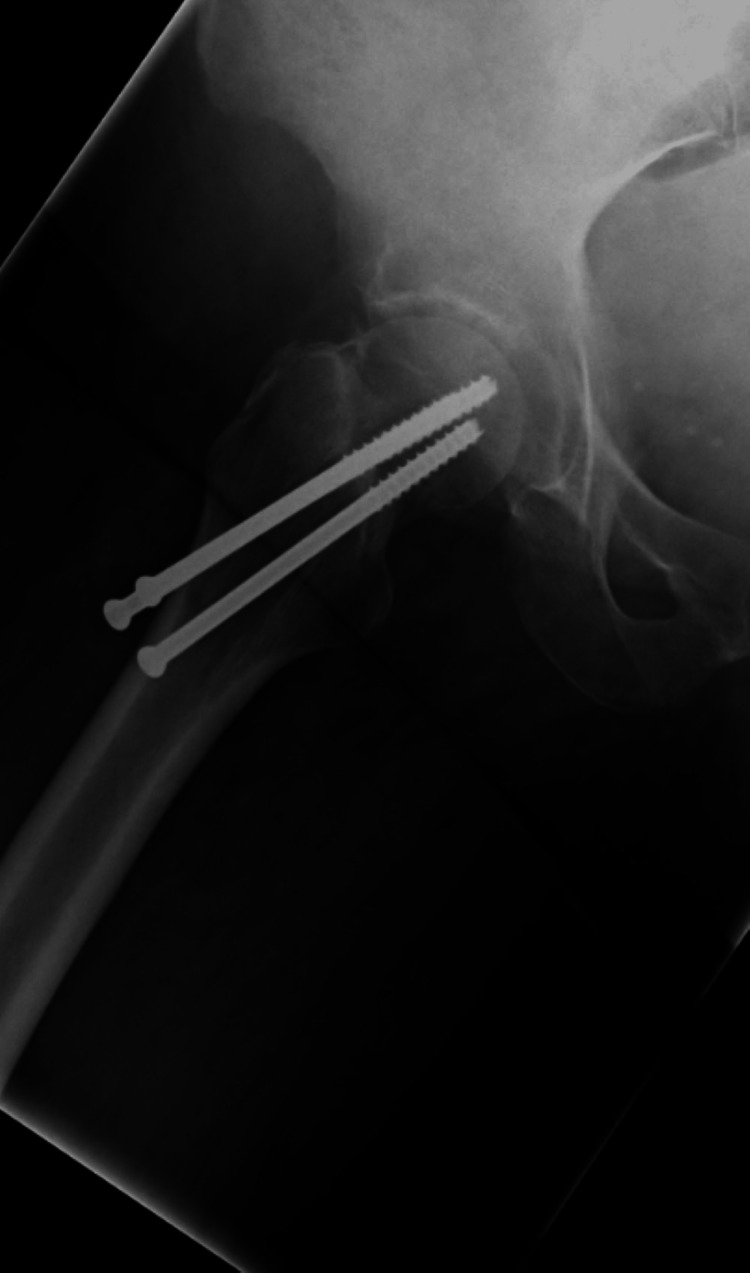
Lateral-view X-ray of fracture non-union
